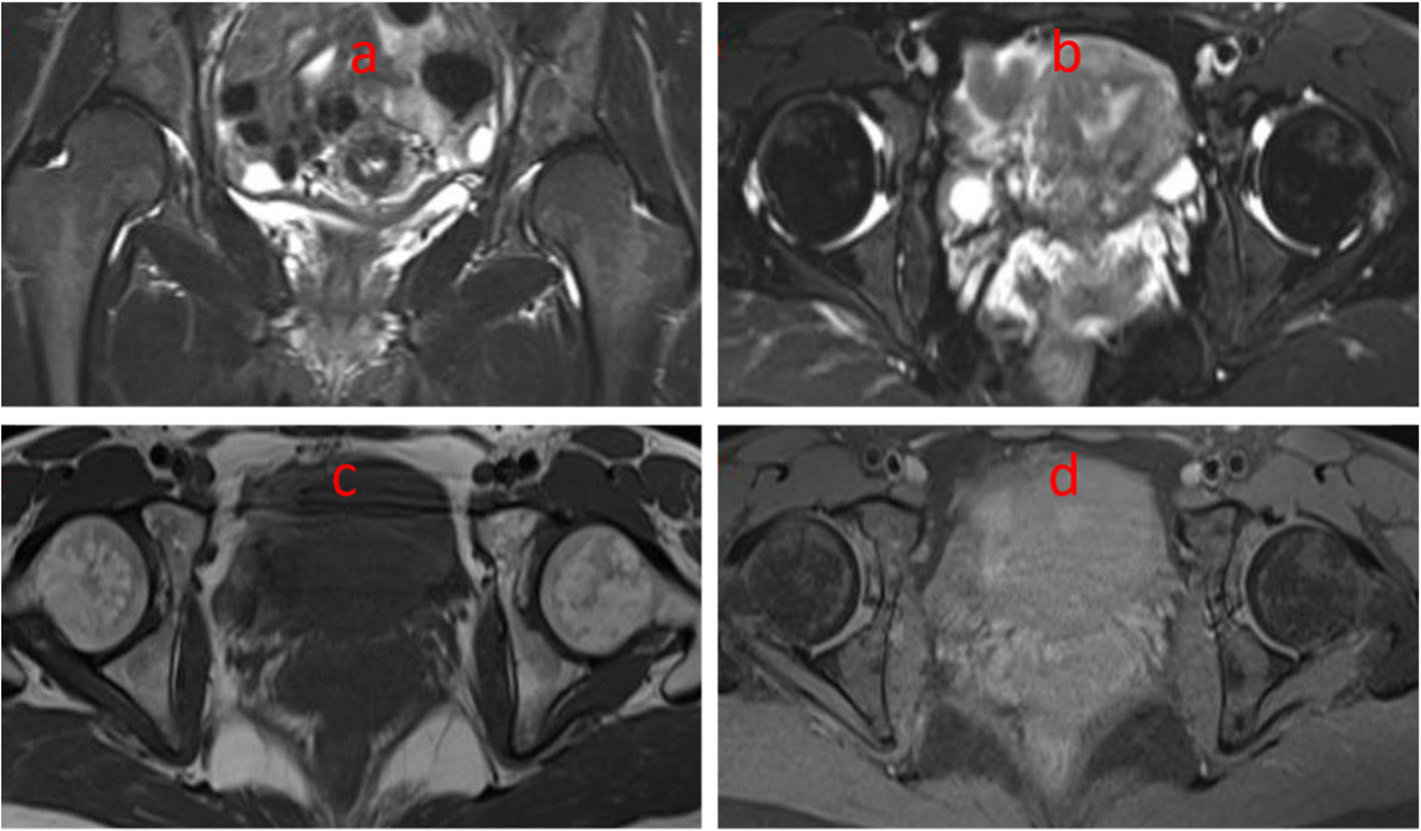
Magnetic resonance images of the hip of a patient with DDH. a T2 and coronal plane. b T2 and transverse plane. c, d T1 and transverse plane
